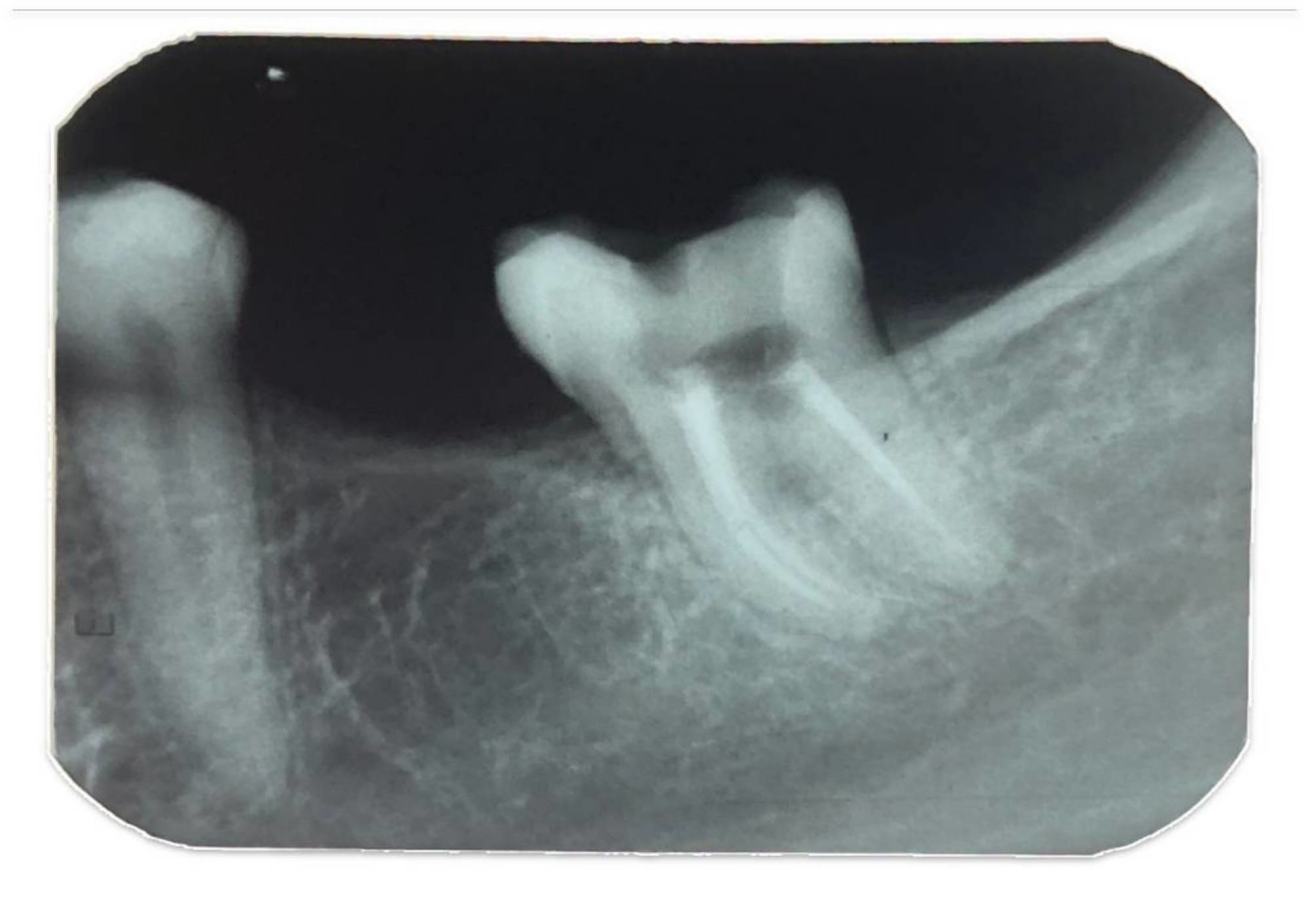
Preoperative Intraoral Radiograph
